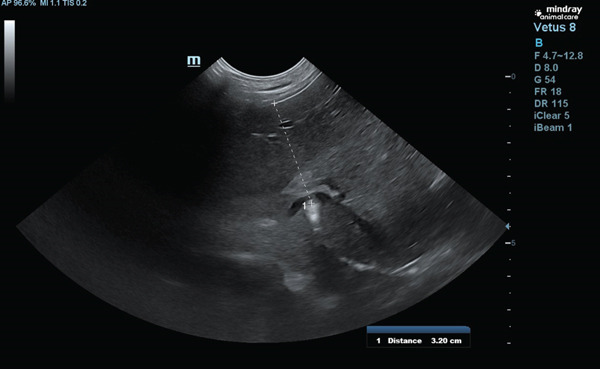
Ultrasound showing longitudinal hepatic approach in the right hypochondrium, with medial angulation of ultrasound probe.
